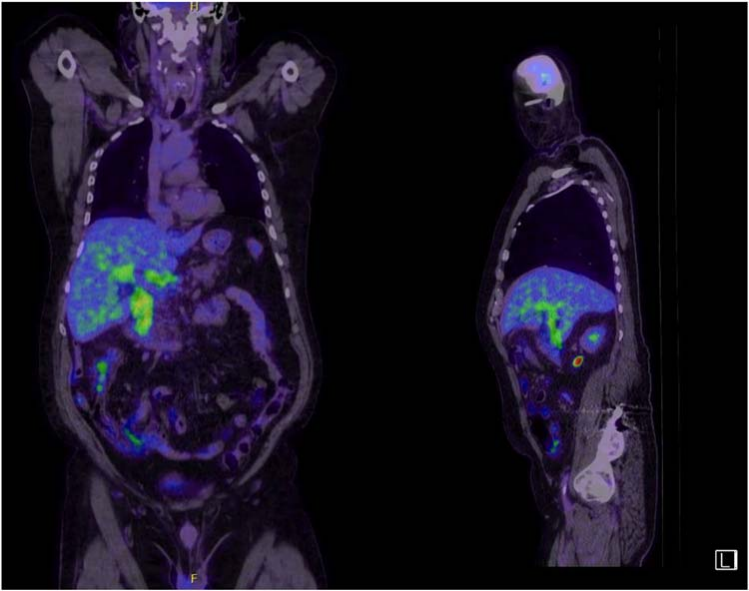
Abnormal FDG uptake on PET scan extending from intrahepatic duct to distal common bile duct with uptake in the tail of pancreas.
